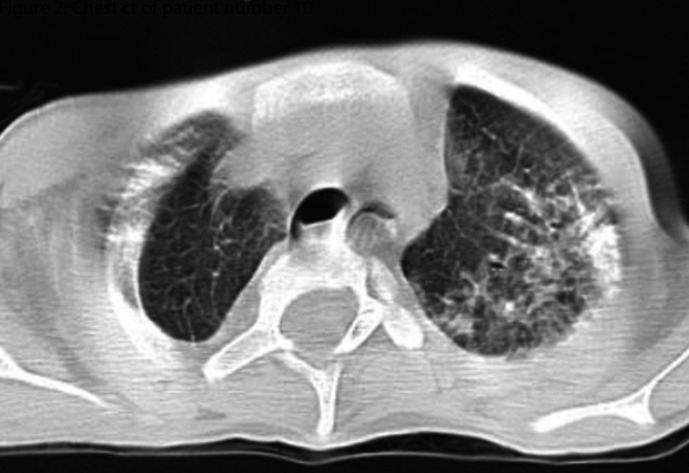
Chest CT of patient number 10.
